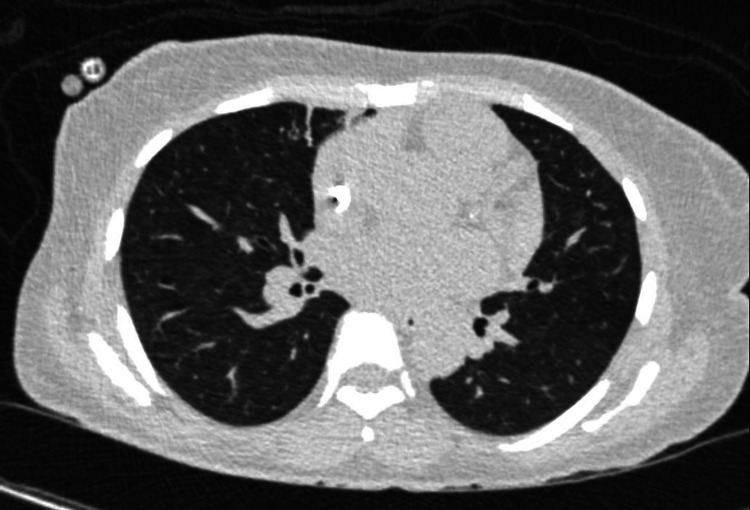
CT Chest CT scan with a focus on branching and ground glass opacity in the right lung apex and the right upper lobe with bronchiectasis
